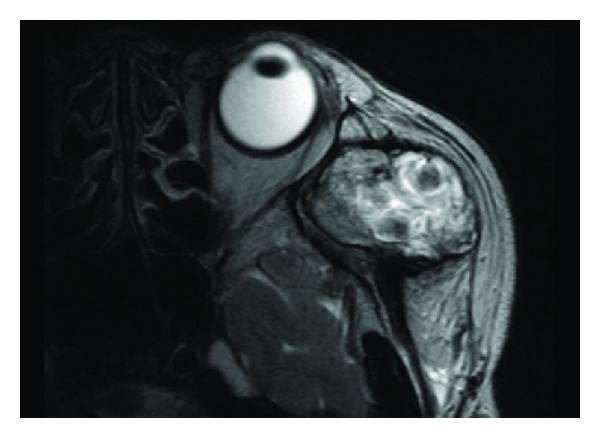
Preoperative MRI on T2-weighted image showing high signal intensity admixed with irregular low signal areas in left temporal fossa.
